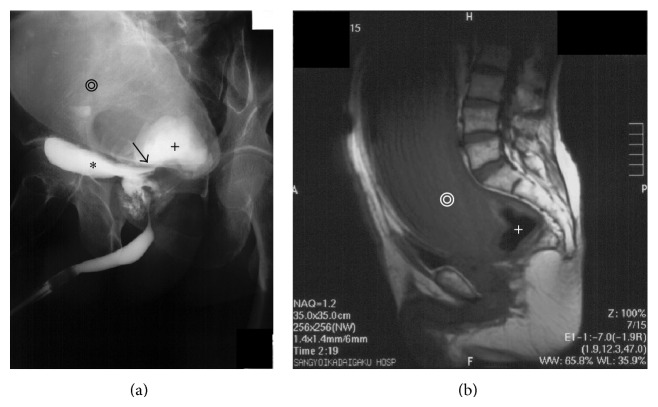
(a) Retrograde urethrography indicated a dilated rectum (black double concentric circles), urinary bladder (black star), and rectourethral fistula between the prostate urethra and lower rectum (black arrow) with stones (black cross). (b) Magnetic resonance imaging (MRI) revealed a megarectum (white double open circles) with stones (white cross).
